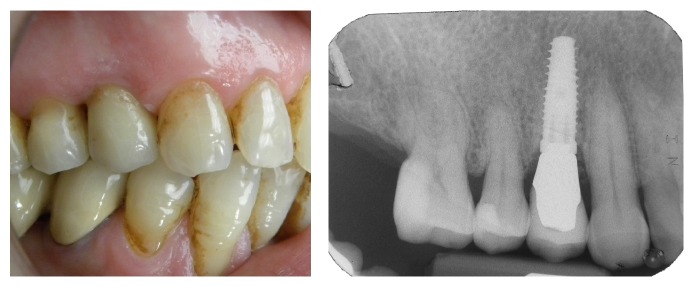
Case 2: definitive prosthetic restoration with periapical radiograph.
